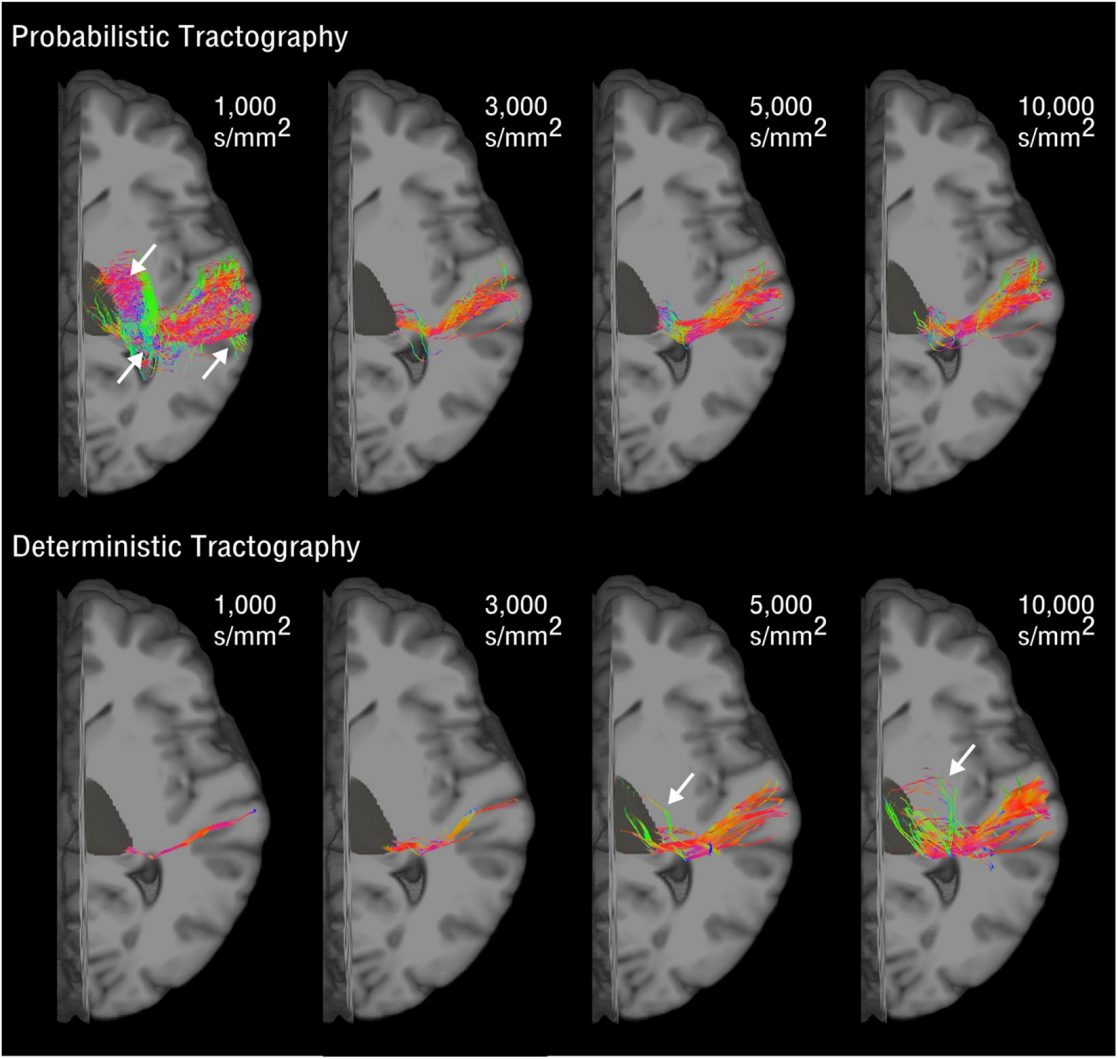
Diffusion MRI Acquisition Parameter Effects on Acoustic Radiation Reconstructions. The image shows the probabilistic (upper row) and deterministic (lower row) tractography reconstruction of the acoustic radiation in the right hemisphere. A representative subject is shown for each diffusion b-value shell and default tractography parameters (angle = 45°, step size = 0.75 *mm*). White arrows point to false positives in the tractography reconstructions. The reconstructed streamlines are shown overlaid on the T1-weighted image of the subject. The streamline colour encodes directionality (anterior posterior: green, superior-inferior: blue, left-right: red). The 3D rendering of the right thalamus is also shown in dark grey.
